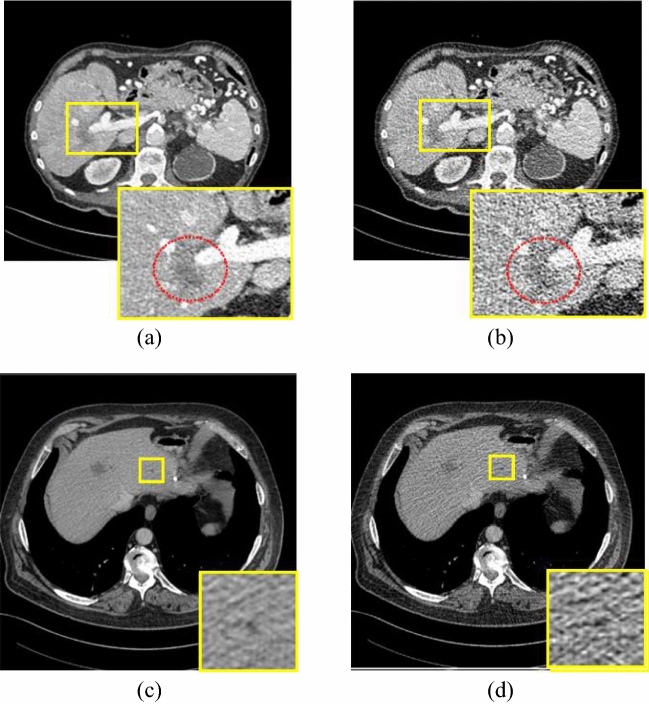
Visuals of CT degradations. a, b Normal dose and quantum noise corrupted abdomen CT image (The metastasis in of liver lesion marked in a red circle is unclear.) [77]; c, d normal dose and quantum noise corrupted abdomen CT images with streak artifacts [73]
